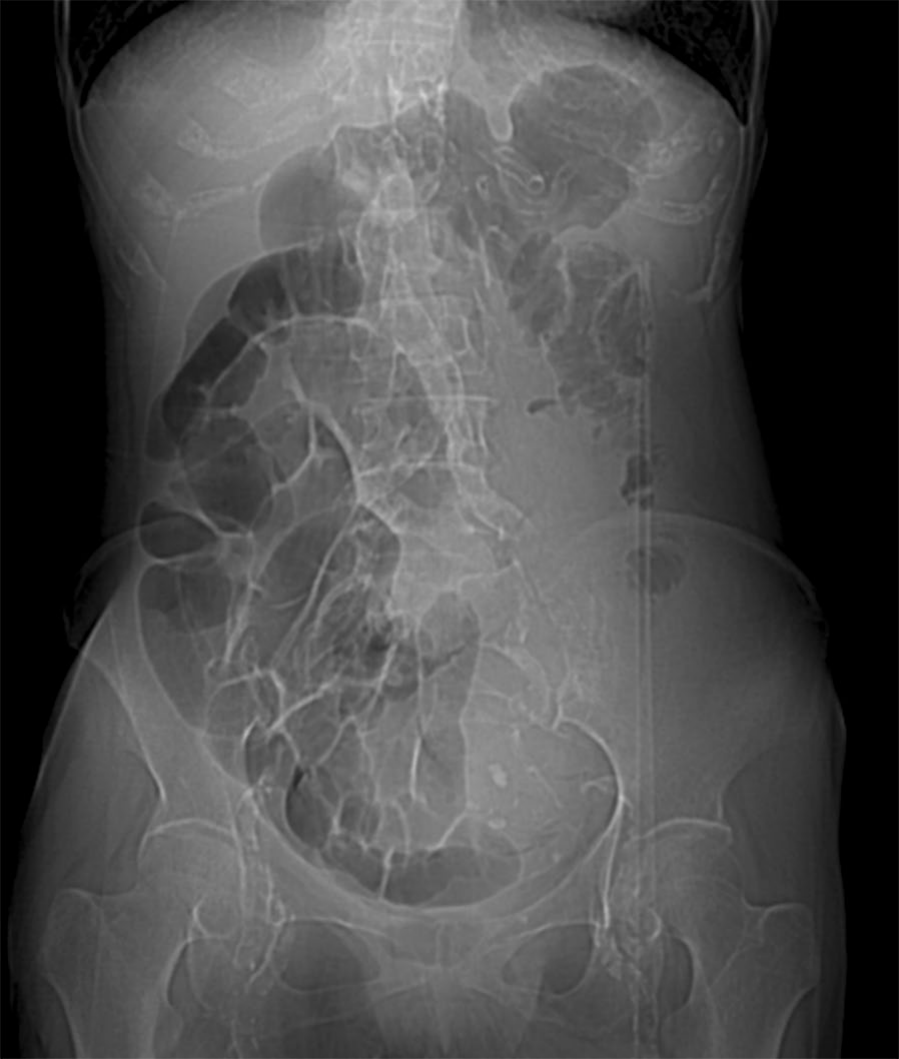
Abdominal computed tomography (CT) scout. Findings suggest intestinal dilation and intestinal gas-like obstruction on the right side and a paucity of gas in the middle to the left side
